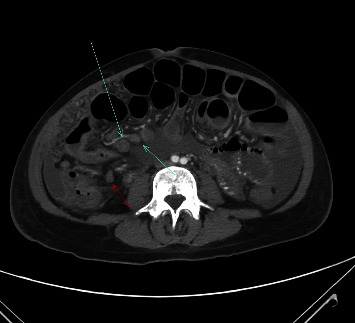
An additional CT image of the abdomen demonstrates mesenteric adenopathy (green and red arrows).
